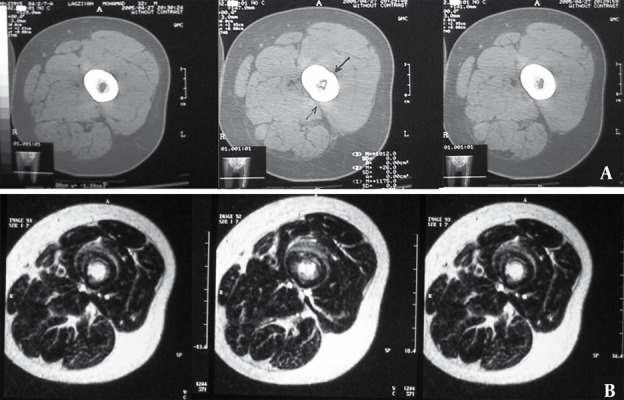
A 25-Year-Old Man Complaining of Pain in Mid FemurA. A, CT scan through mid-femur. Nidus is visible in the medulary region only in one of the cuts. B, MRI of the same patient reveals distinct hypersignal nidus in the medulary region in T2-W sequence in several cuts.
